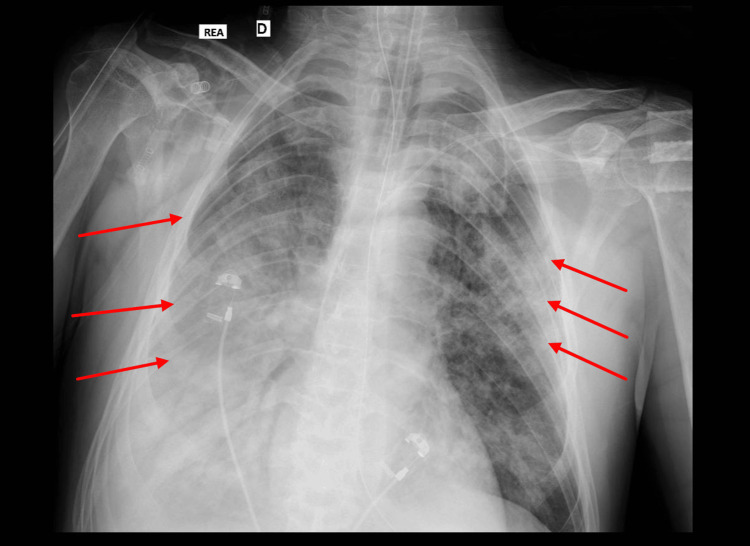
Chest X-ray showing bilateral pulmonary infiltrates with the right white lung
